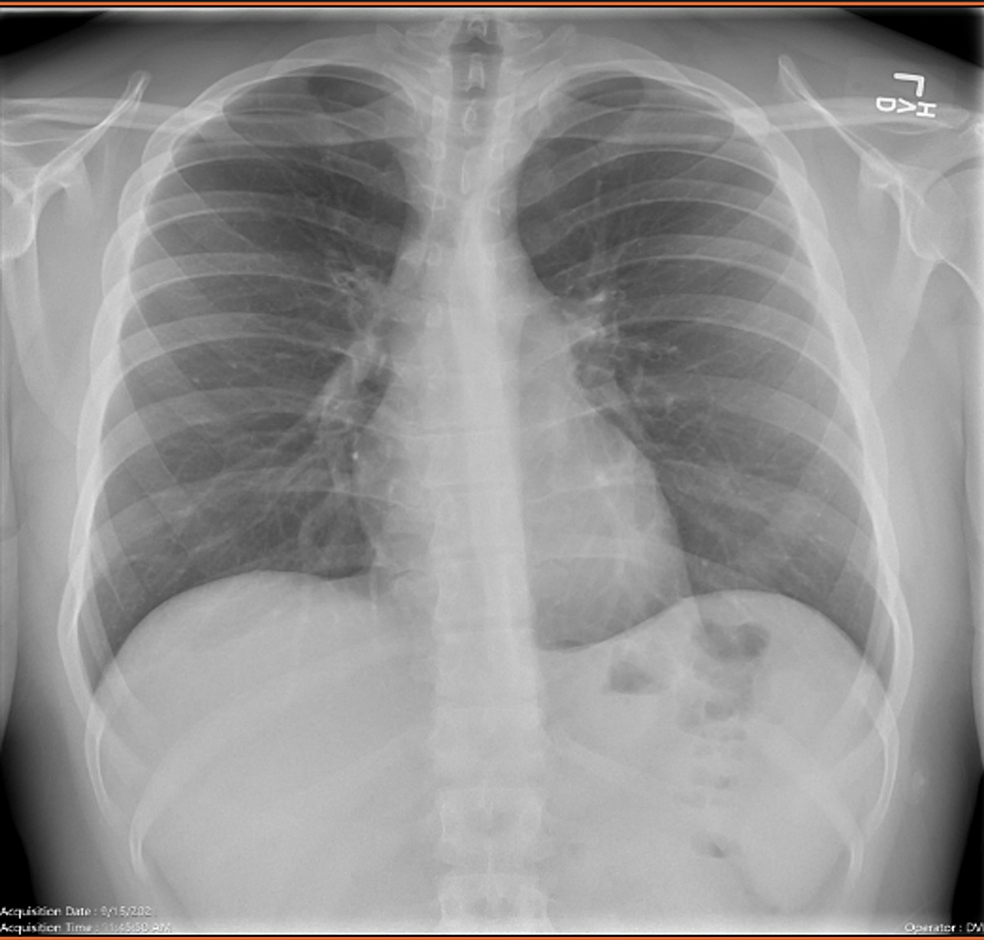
Chest X-ray showing normal cardio mediastinal silhouette. The pulmonary vasculature is unremarkable. ​No focal airspace opacity, pneumothorax, or pleural effusion is seen​.
